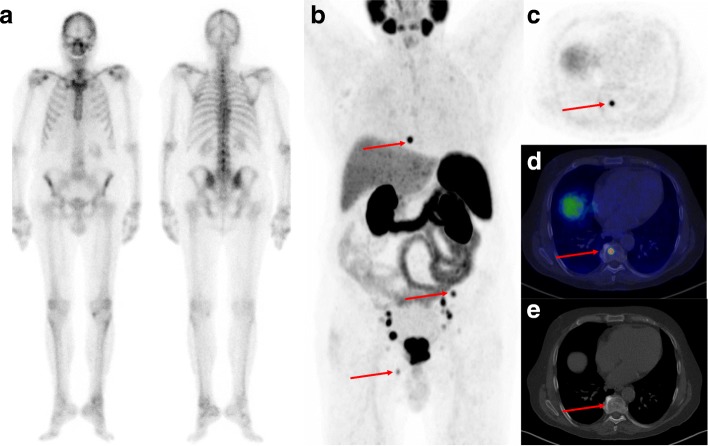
Example of a patient (PSA 44 ng/mL, Gleason score 9, T3) classified as M0 according to initial BS as shown in anterior (a) and posterior projection (b). The patient was referred for ^68^Ga-PSMA-11 PET/CT due to high-risk prostate cancer. The maximum intensity projection (MIP) of the ^68^Ga-PSMA-11 PET (c) revealed several lesions with avid ^68^Ga-PSMA-11 uptake, including three bone metastases marked with arrows (Th8, left iliac bone and right pubic bone). The axial ^68^Ga-PSMA-11 PET image of the lesion in Th8 is shown in c with a fused ^68^Ga-PSMA-11 PET/CT image shown in d and only a slight sclerotic change in the axial CT image (e). BVC confirmed M1 status
